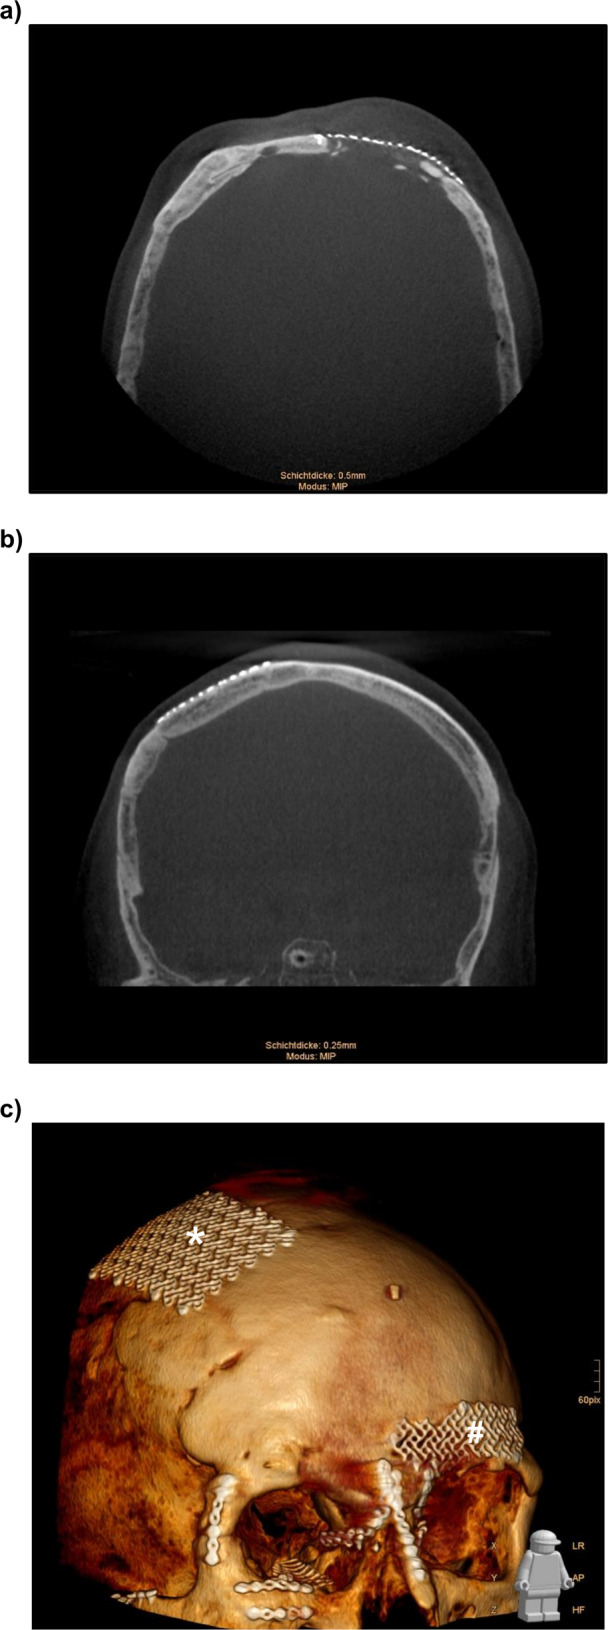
CT scan of a patient 20 years after fracture treatment of the skull [(a): axial slice of a frontal sinus fracture; (b) coronal slice of a fracture of the parietal bone]. No proper healing can be observed in the frontal sinus area, using only a simple covering with a titanium mesh. c 3D reconstruction of the same CT scan showing the exact location of the two fracture regions in the frontal [#] and parietal [*] region
